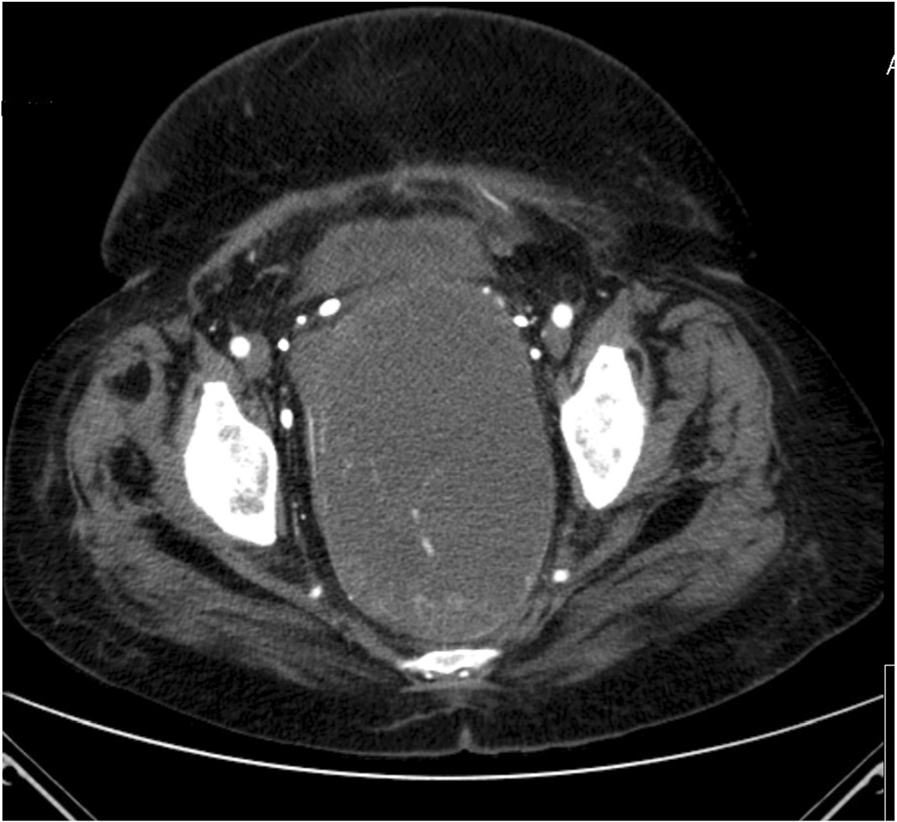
Preoperative and postembolization CT showing partial devascularization
